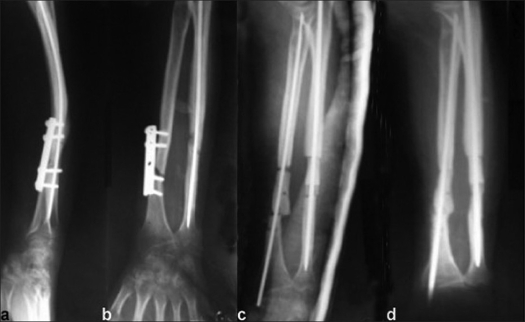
X-ray of right forearm lateral (a) and anteroposterior (AP) view (b) of a case of 10-month old ununited fracture both bones of the forearm with implant *in situ* (plate). AP view (c) shows fracture fixation with intramedullary nail and interposed tricorticocancellous strut bone graft. 20 weeks follow-up AP X-rays (d) shows fracture union with incorporation of bone graft
